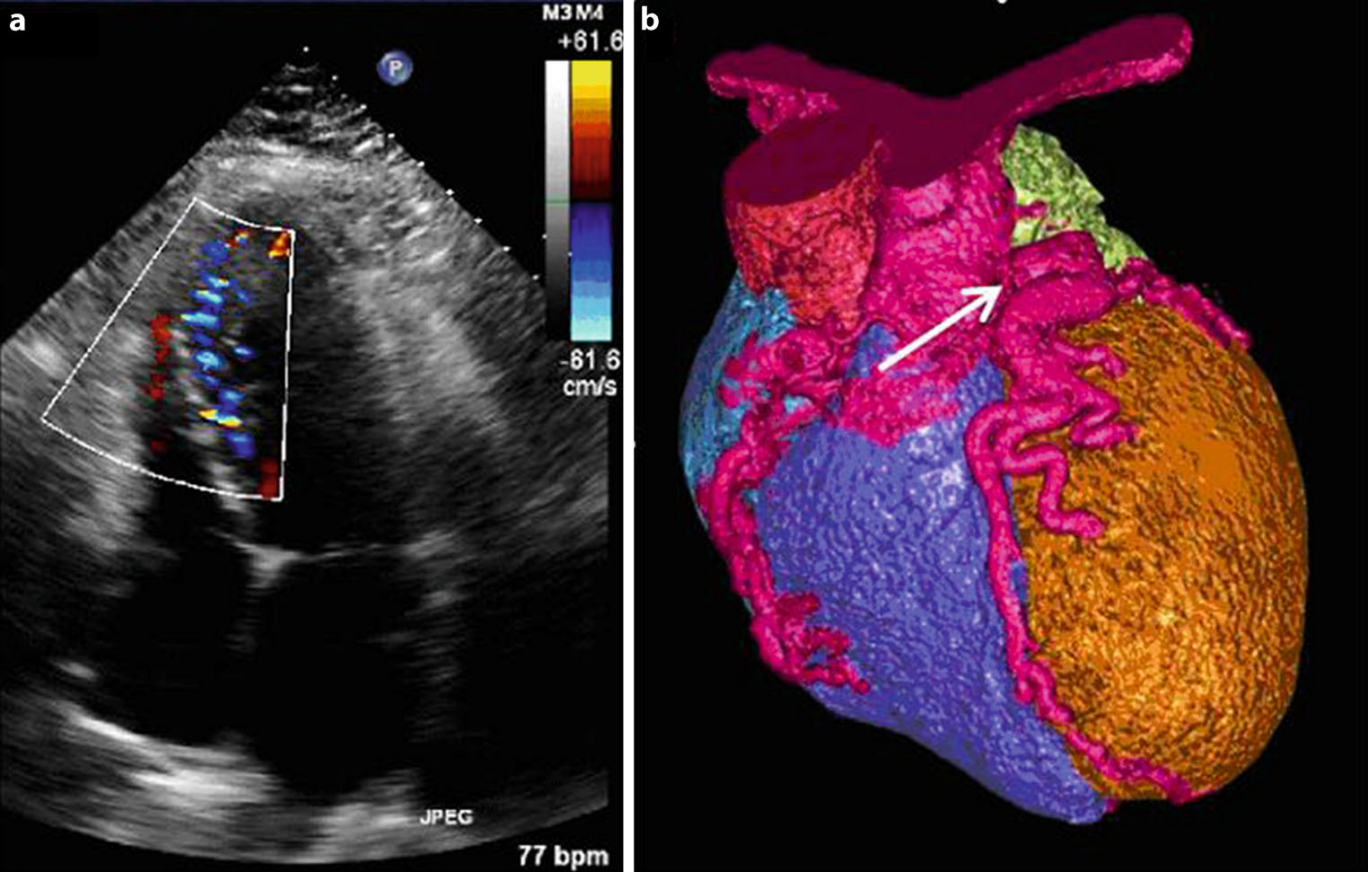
a Apical four chamber view showing extensive septal collaterals with colour Doppler. b Volume-rendered cardiac CT showing the left coronary artery originating from the pulmonary artery
